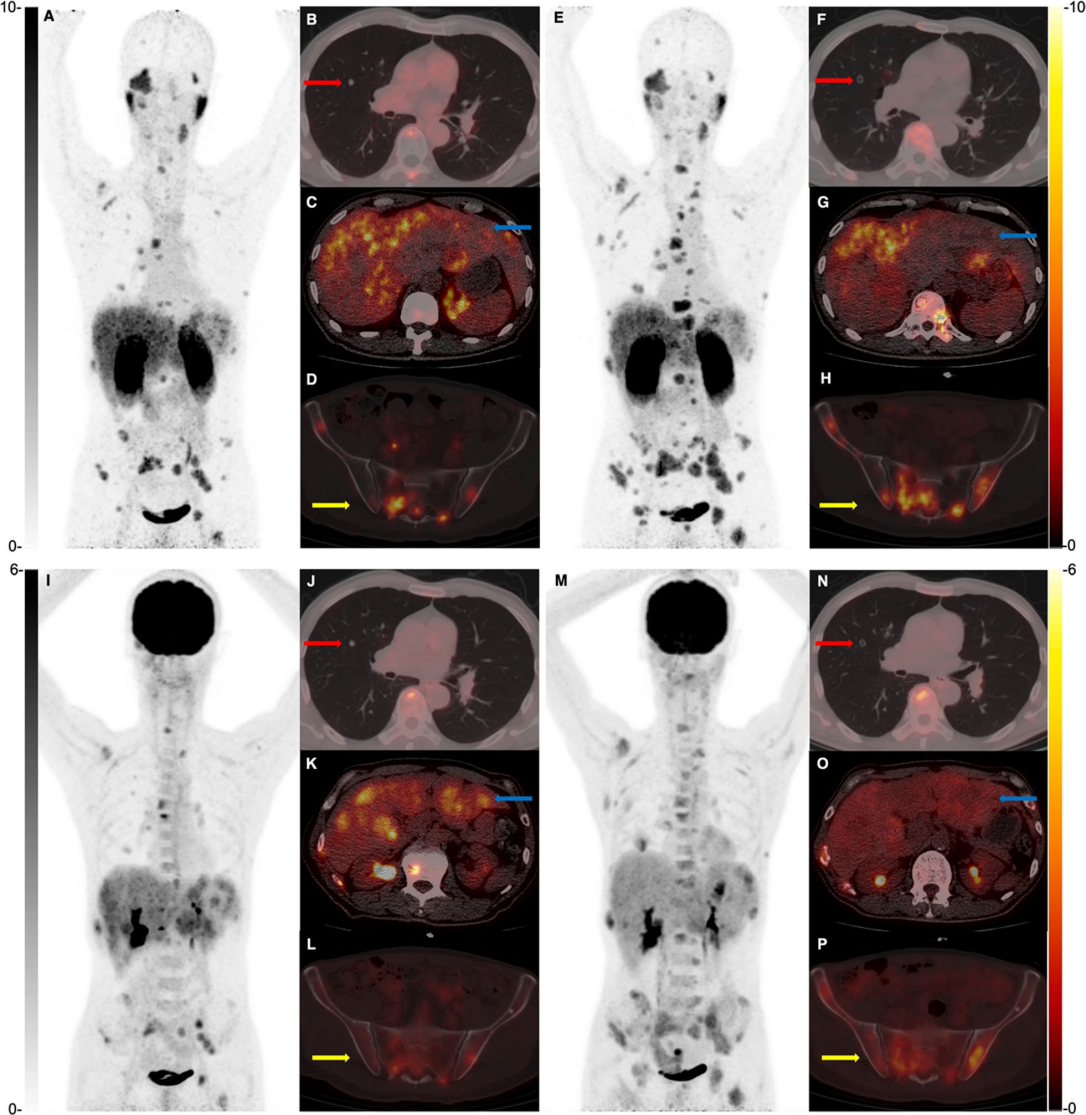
A 56-year-old male patient was diagnosed with local recurrence and multiple metastases 12 months after surgical removal of nasal ACC. Pre-therapy ^68^ Ga-PSMA-617 (A–D) and ^18^F-FDG PET/CT (I–L) depicted neoplasm recurrence, multiple liver, bone, and lung metastases. ^68^ Ga-PSMA-617 PET/CT (E–H) and ^18^F-FDG PET/CT (M–P) reexamination at 8 weeks after the 1st cycle of PSMA RLT revealed the decreased SUVmax of lung metastases (red arrow) and liver metastases (blue arrow); and CT showed liquefied necrosis occurred inside the lung nodule (red arrow). The uptakes of bone metastases (yellow arrow), however, were significantly increased
